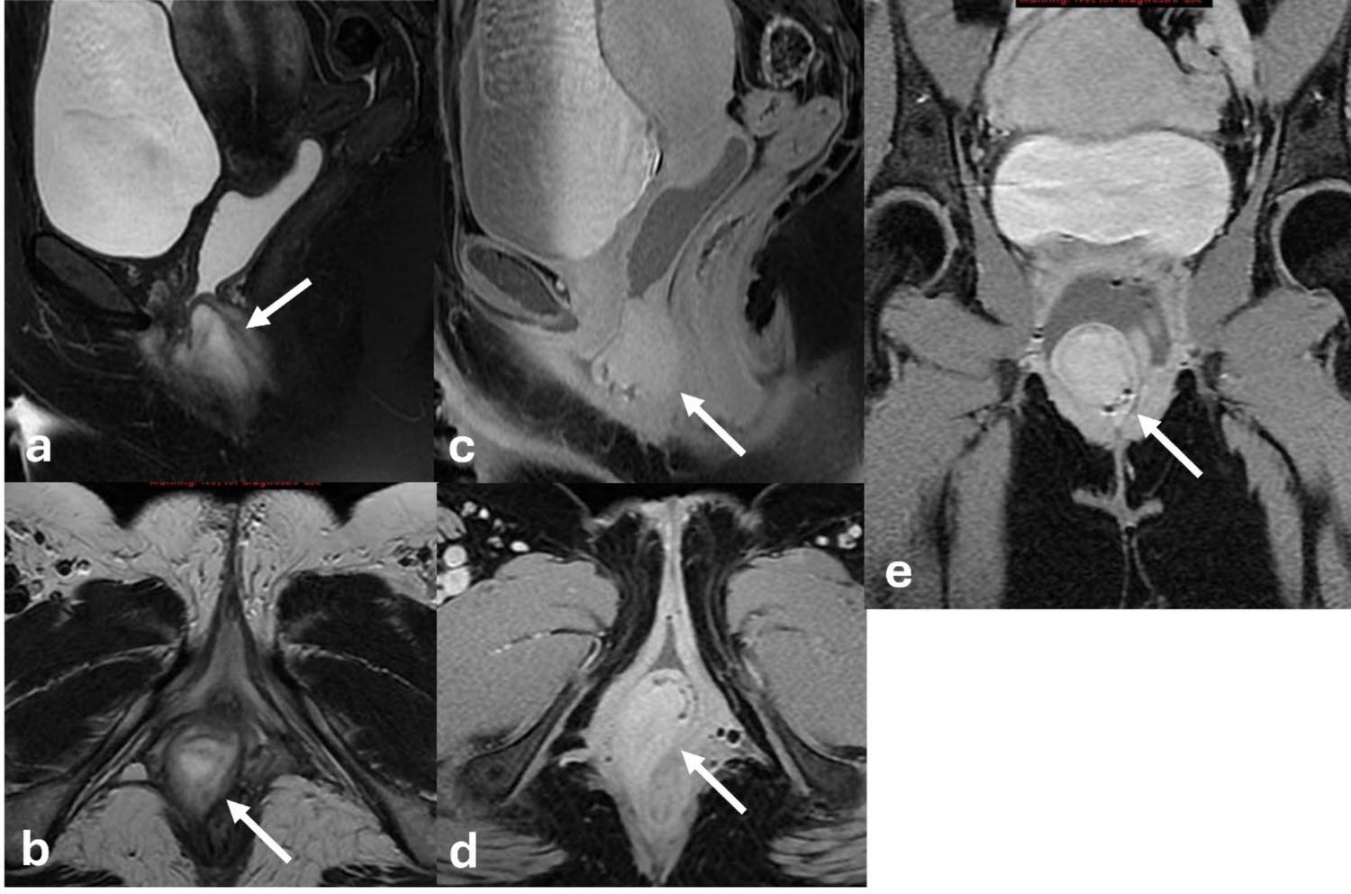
43-Year-old female presenting without significant past medical history presenting with vaginal discomfort without associated bleeding or discharge. A right posterior vaginal mass was discovered on physical exam. MRI was obtained for further characterization. This individual underwent subsequent surgical resection with pathology confirming aggressive angiomyxoma. Sagittal fat-saturated T2WI and axial non fat-saturated T2WI of the pelvis following administration of vaginal gel demonstrate a T2 heterogeneous lesion (arrow) centered in the right lateral aspect of the vagina extending to the introitus. The lesion demonstrates uniform enhancement on sagittal (a), axial (d) and coronal (e) post-contrast T1WI
